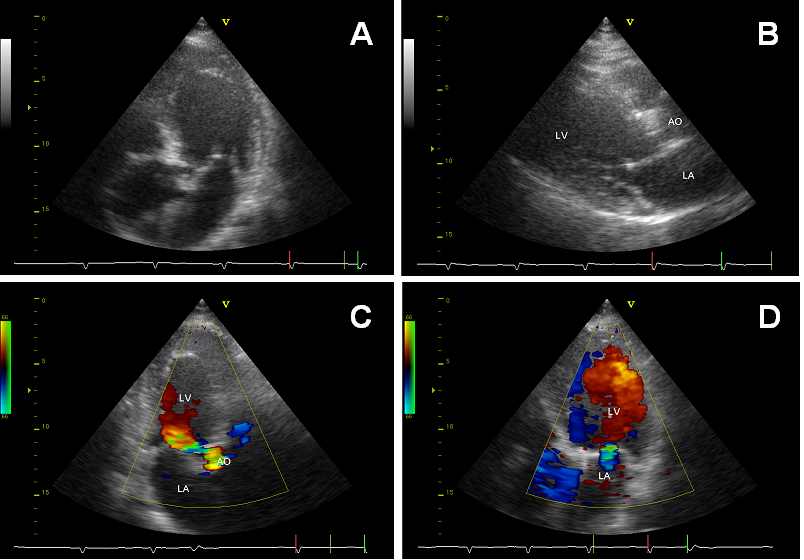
Echocardiogram of the patient. (A) Apical four-chamber view with aorta. (B) Parasternal long axis view with the root of the aorta, left atrium and left ventricular outflow tract. Both images show moderate calcification of the aortic valve and slight calcification at the anterior leaflet of the mitral valve. (C) Apical two-chamber view with aorta. Colour flow mode indicates moderate aortic regurgitation. (D) Apical four-chamber view. Colour flow mode indicates mild mitral regurgitation.
